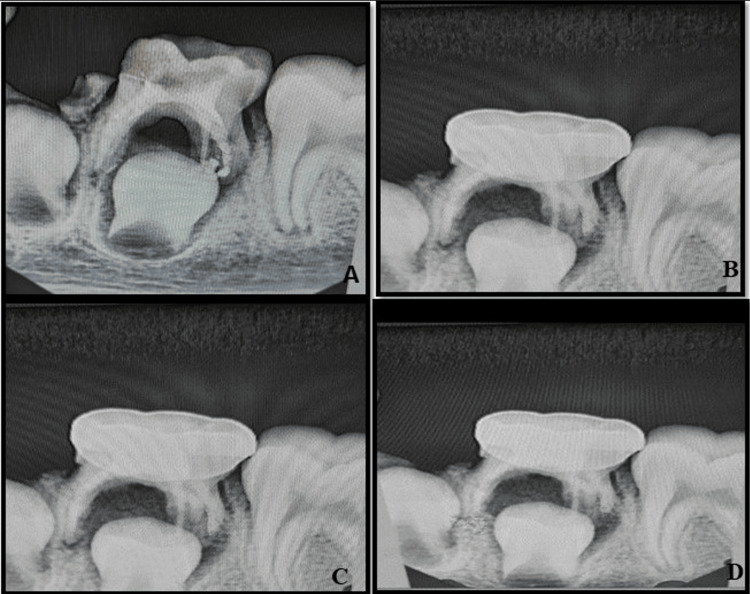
Radiographic assessment of Endoflas (A) Immediate postoperative radiograph showing overfilling at root apex. (B) Postoperative radiograph at three months. (C) Postoperative radiograph at six months. (D) Postoperative radiograph at nine months.
